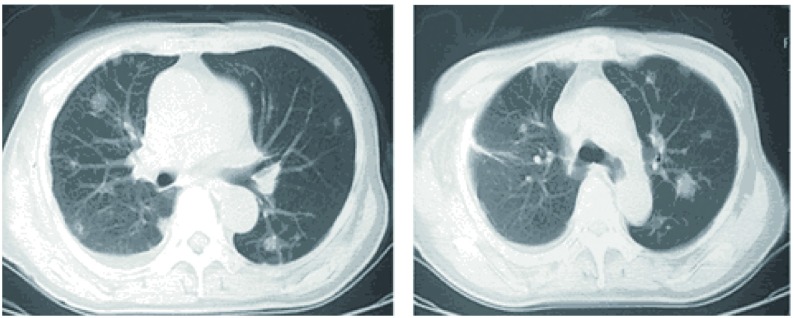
术后胸部CT检查示双肺剩余结节未见明显变化 CT scans of the chest show that the left nodules in both lungs has not change obviously
